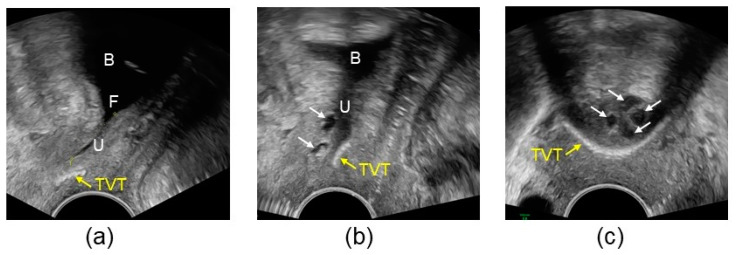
Pelvic floor sonography: Combined effect of bulking agent (Bulkamid^®^) and a sling. (a) Recurrence after TVT insertion, situation before Bulkamid^®^ injection. In the sagittal view, funneling is visible at straining, the position of the TVT is too distal; (b) Continence after subsequent Bulkamid^®^ insertion. At straining, the Bulkamid^®^ depot is pressed towards the TVT. Together, they achieve urethral continence; (c) Axial view, same patient: the four Bulkamid^®^ depots are localized in one plane around the urethra to render continence. Due to coaptation, the urethral lumen itself is closed while funneling is no longer present. B: bladder; F: funneling; U: urethra; white arrows point to Bulkamid^®^ depots; yellow arrows point to TVT.
